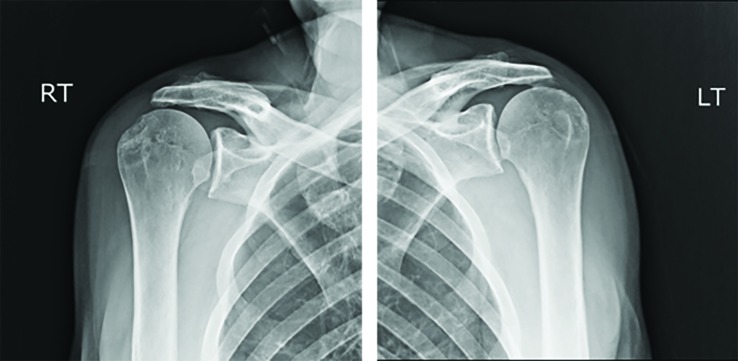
Radiography taken 2-weeks after discharge, which demonstrates bilateral high-riding humeral heads with concentrically reduced shoulder joints
